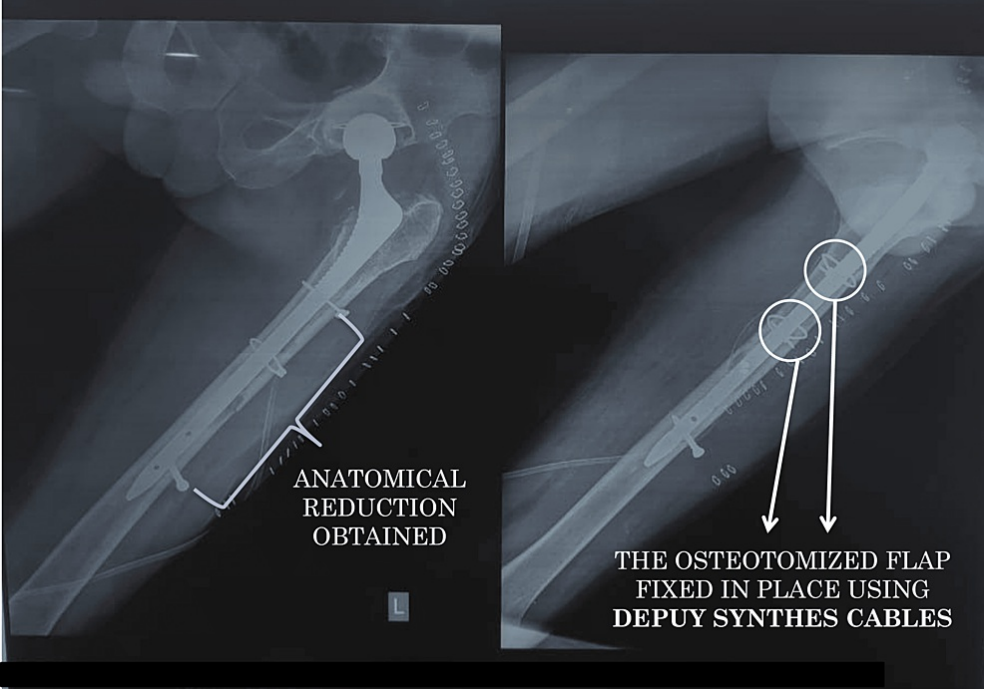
Postoperative plain radiograph of the left femur Anatomical reduction was achieved, and the osteotomy flap was fixed in place with DePuy Synthes Cables
